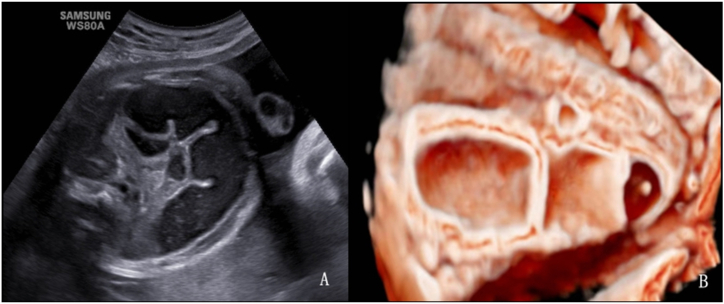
Fetus at 27 weeks and 3 days of gestation with small intestinal dilatation. Postnatal surgery confirmed ileal atresia. A. 2D ultrasound shows intestinal dilatation, with localized distribution of small bright spots within the intestinal lumen. During peristalsis, the walls of two adjacent intestines come into contact and protrude into the intestinal lumen. B. In 3D volume imaging in grayscale mode, the dilated intestinal segment is identified as the small intestine with the smooth inner wall.
